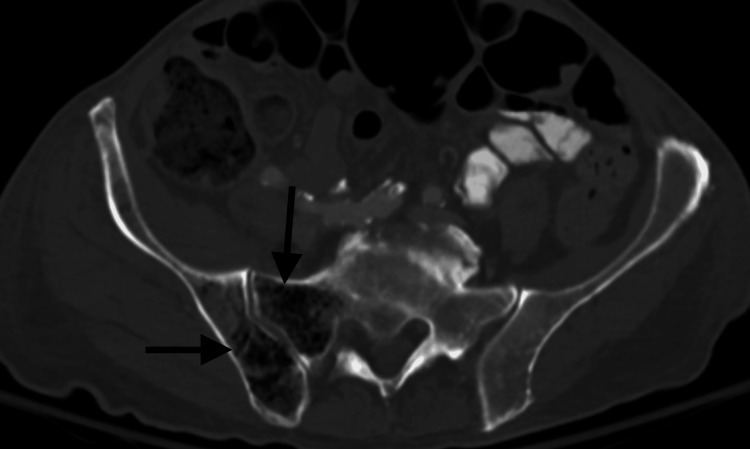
Emphysematous osteomyelitis of the sacrum and pelvis. Axial CT abdomen/pelvis in an 80-year-old Caucasian man presenting with worsening generalized weakness, dyspnea, and tachycardia with black arrows demonstrating emphysematous osteomyelitis of the right iliac bone and right sacroiliac joint.
